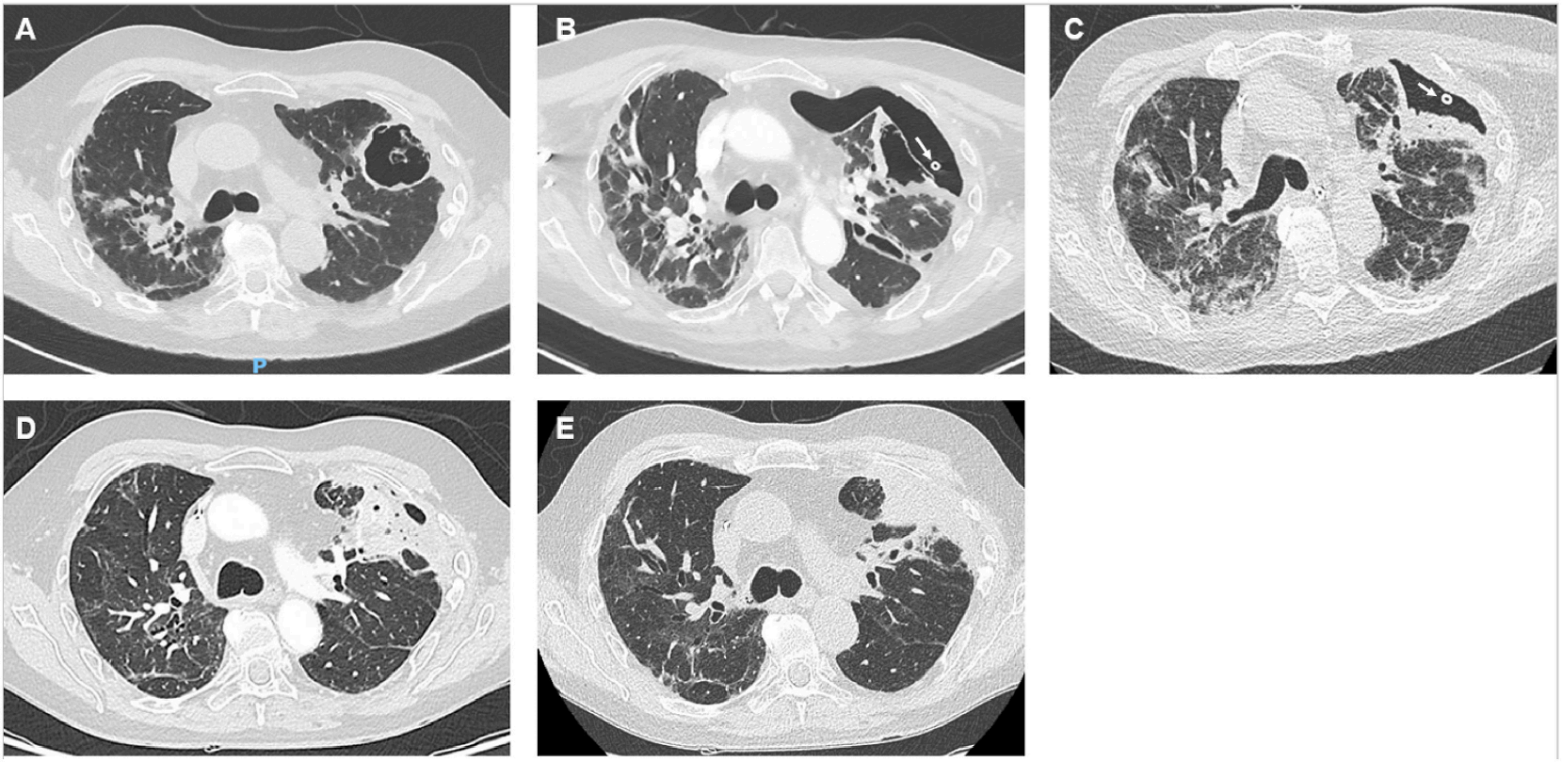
CT scan showing A: Aspergillosis subpleural cavitation before pneumothorax; B: left pneumothorax with alveolar-pleural fistula resulting from the rupture of the pulmonary cavitation (white arrow indicates the cross-section of the drain catheter); C: left lung expansion after EBV placement (white arrow indicates the cross-section of the drain catheter); D and E: aspergillosis cavitation disappearing giving place to a residual consolidation.
